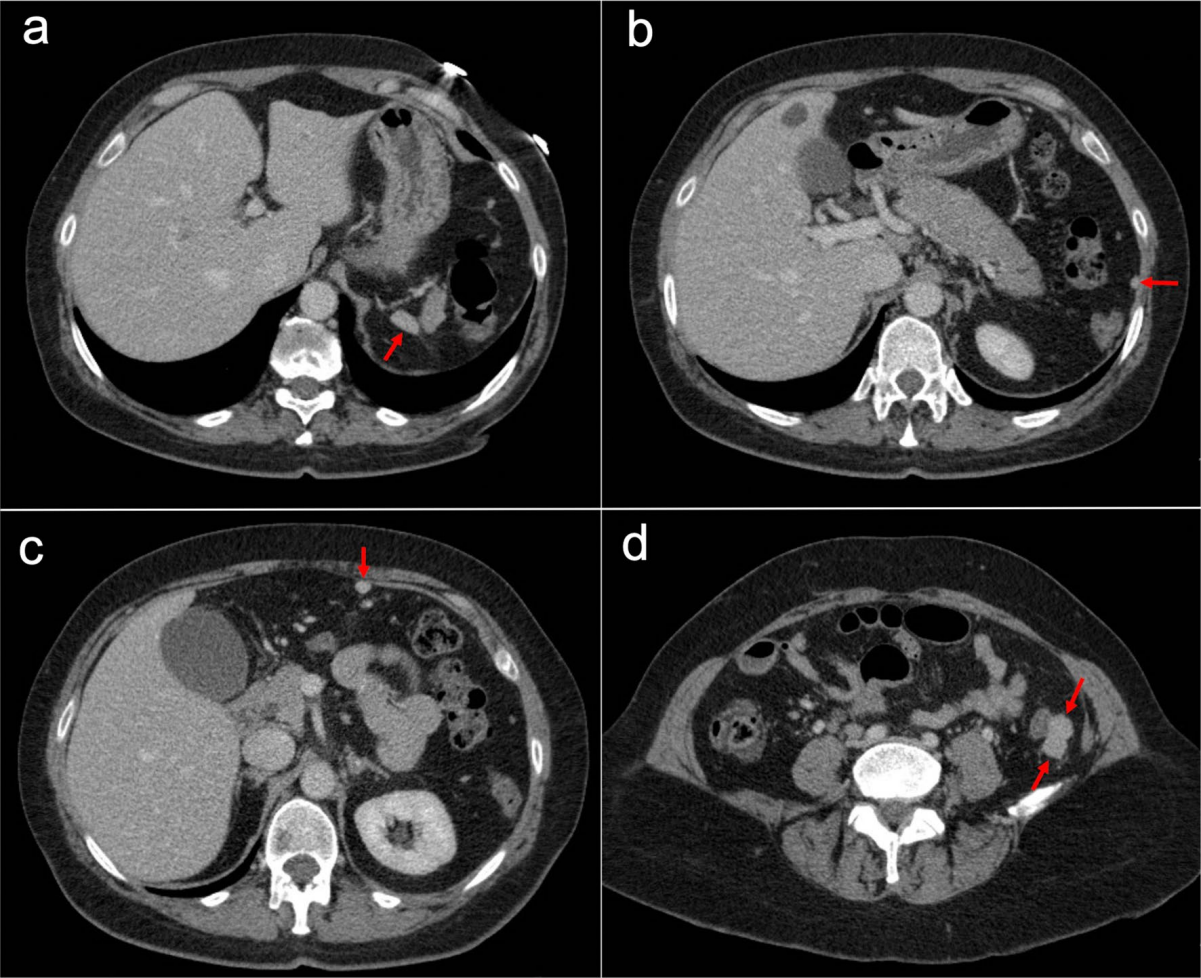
Incidental splenosis in a 50-year-old woman with history of previous splenectomy and abdominal discomfort. a–d Axial CT images in the portal phase show solid peritoneal nodules in the splenic fossa and left paracolic gutter, with homogeneous contrast uptake (red arrows). The distribution and characteristics of the lesions was consistent with splenosis implants and there were no other pathological findings in the rest of the abdomen. No further imaging was considered necessary
